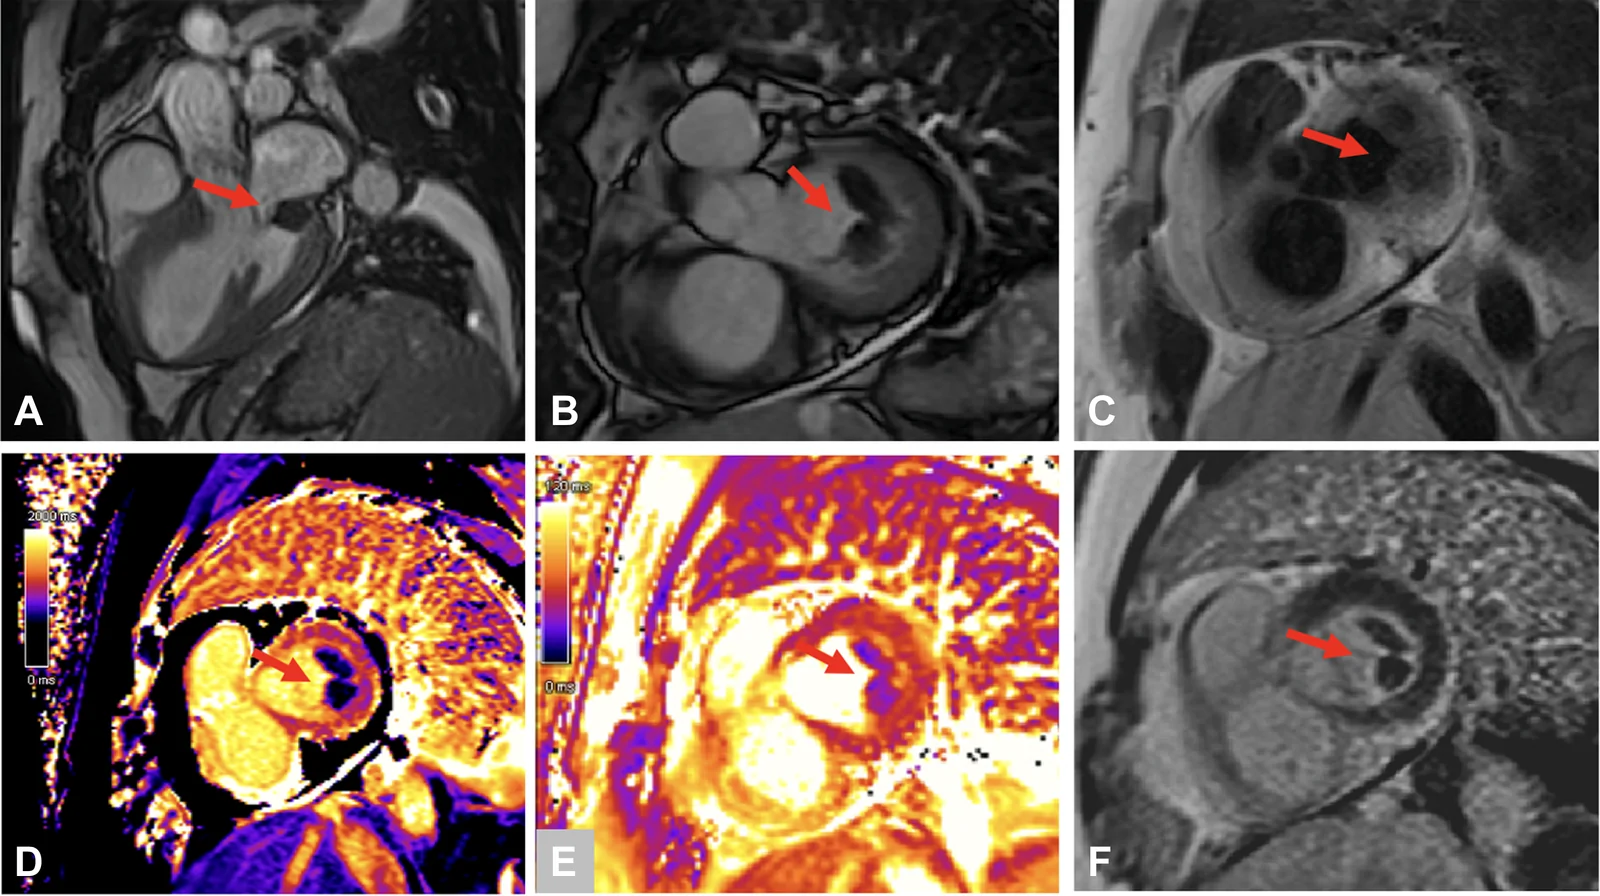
Cardiac Magnetic Resonance Balanced steady-state free precession cine sequences in the 3-chamber view (A) and short-axis view (B) demonstrate a hypointense mass with well-defined borders adjacent to the posterior mitral annulus (red arrow). T1-weighted turbo spin-echo sequence in the short-axis view (C) shows the same hypointense mass (red arrow). On parametric mapping, the lesion exhibits low native T1 and T2 values on T1 mapping (D) and T2 mapping (E), respectively. Phase-sensitive inversion recovery late gadolinium enhancement imaging (F) reveals an enhanced rim surrounding a nonenhanced core.
